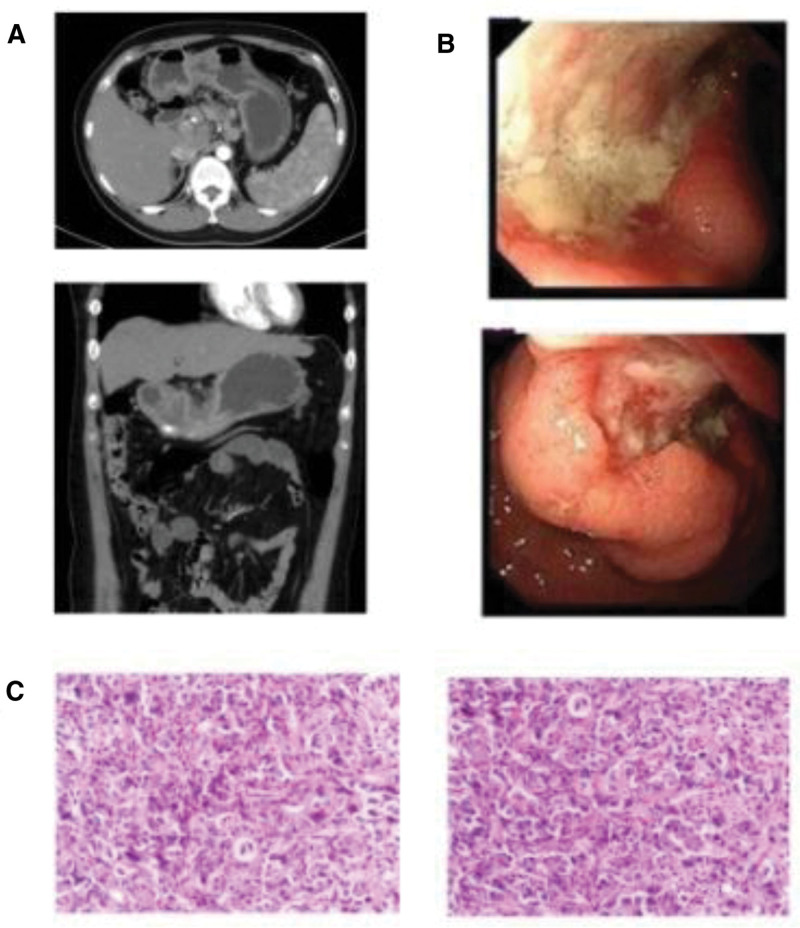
(A) Computed tomography before surgery; (B) gastroscopy showed advanced gastric cancer; (C) pathological examination.
